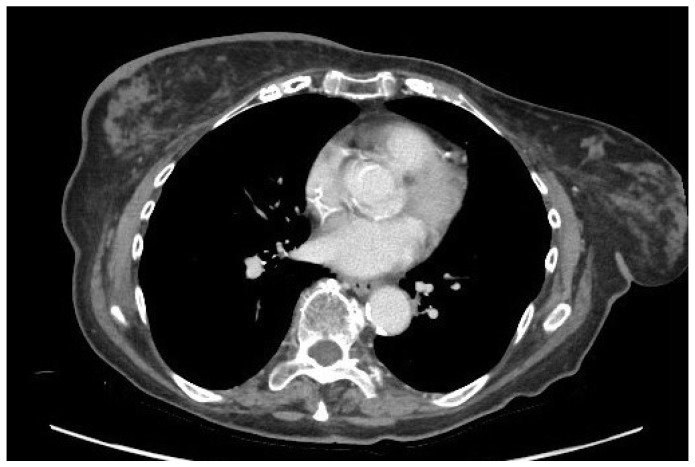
A 75-year-old patient with a history of lobular invasive carcinoma of the right breast treated with quadrantectomy and radiotherapy. Mediastinal window of a follow-up CT scan shows skin thickening of the right breast.
